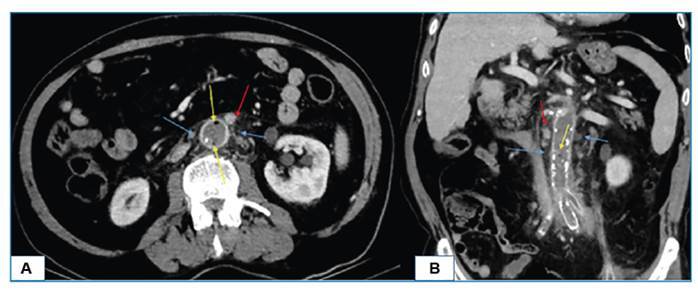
Axial (A) and coronal (B) plane abdomen CT shows occlusion of the distal abdominal aorta, perivascular fat stranding (blue arrows), vessel wall gas formation (yellow arrows), and several lymph nodes (red arrow).
